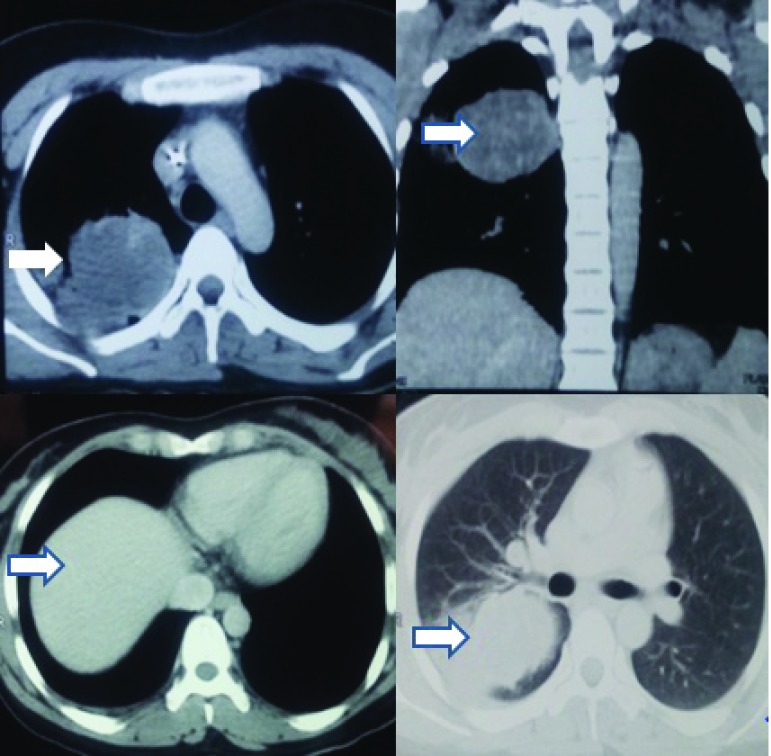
CT chest confirming presence of an enhanced and highly vascular lesion in the upper lobe of the right lung.
